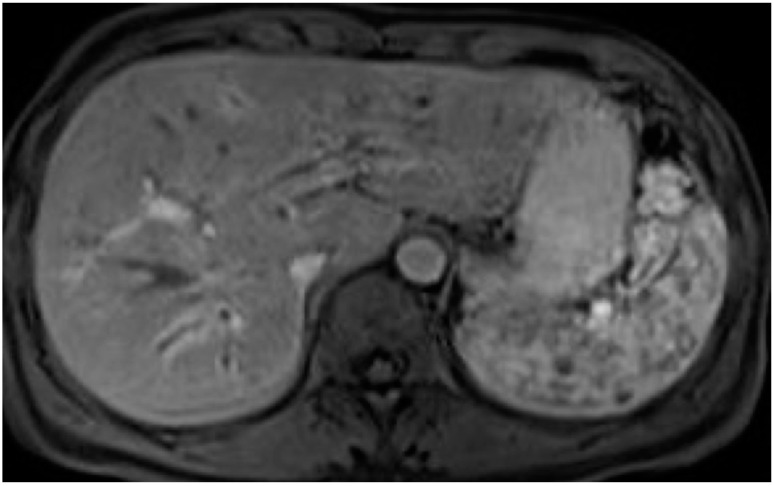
Biliary wall thickening and mural enhancement of the intrahepatic biliary ducts after contrast. T1-weighted sequences after Gd-EOB-DTPA injection.
